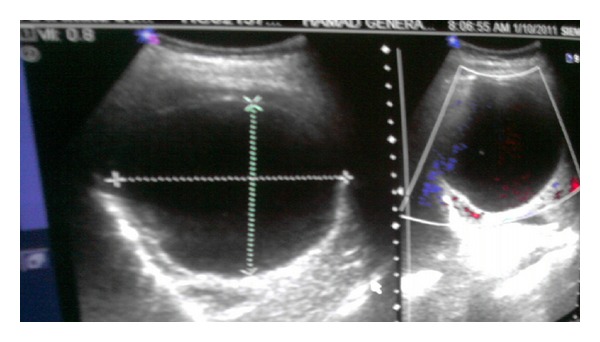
Abdominal ultrasound revealed a well-defined cystic lesion in the right liver lobe, likely hydatid cyst.
